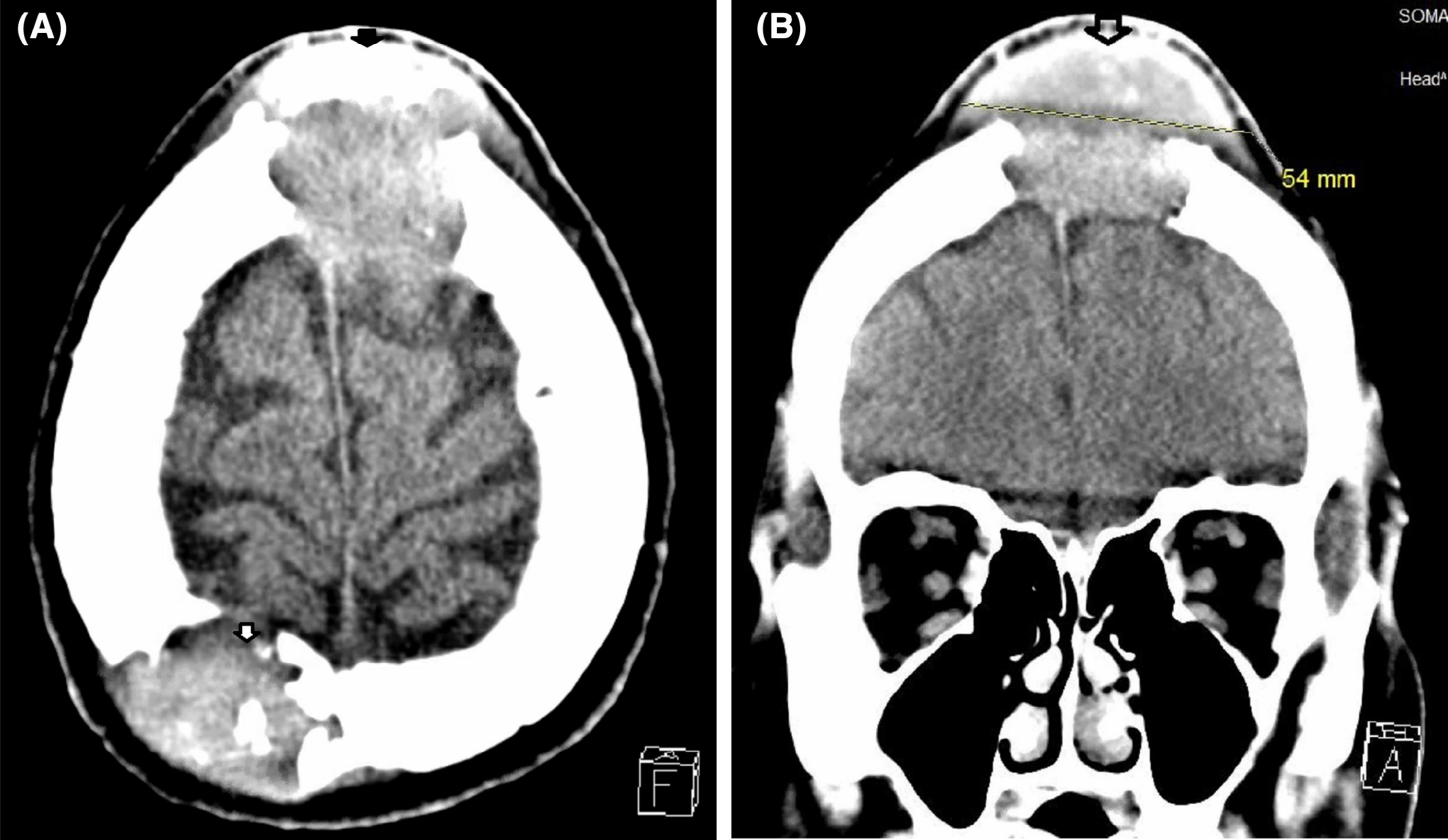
(A) Preoperative head CT scan axial view depicting a right sided parietal extracalvarial metastatic lesion. (B) Preoperative head CT scan coronal view depicting a midline large extracalvarial metastatic lesion over the Bregma.
